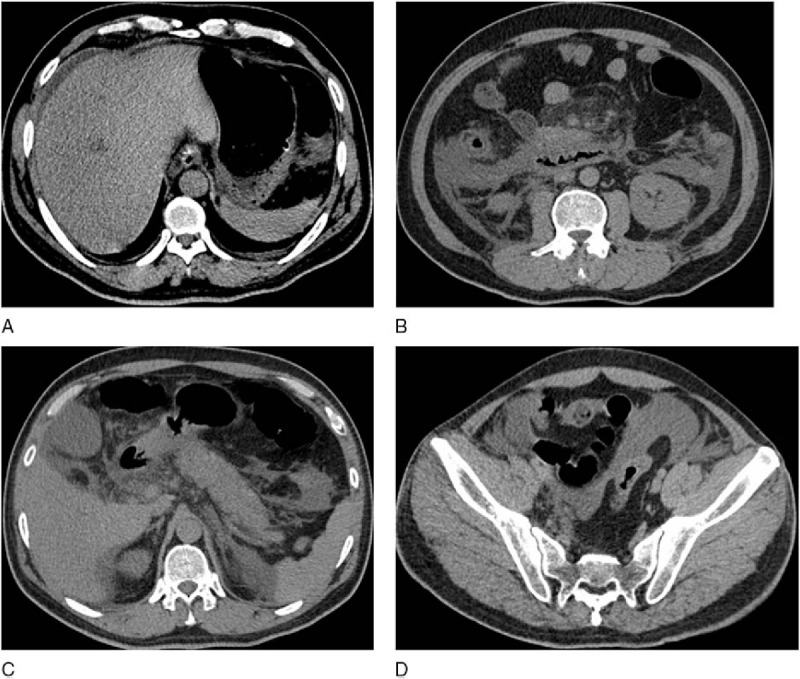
Transverse unenhanced abdominal CT scans of a 51-year-old man with AP who was discharged from the hospital 48 days after admission and had an EPIC score of 6. (A) Unilateral pleural effusions. (B) Bilateral retroperitoneal inflammation and mesenteric inflammation. (C and D) Ascites in perisplenic, perihepatic, interloop, and pelvic locations. AP = acute pancreatitis, EPIC = extrapancreatic inflammation on computed tomography.
